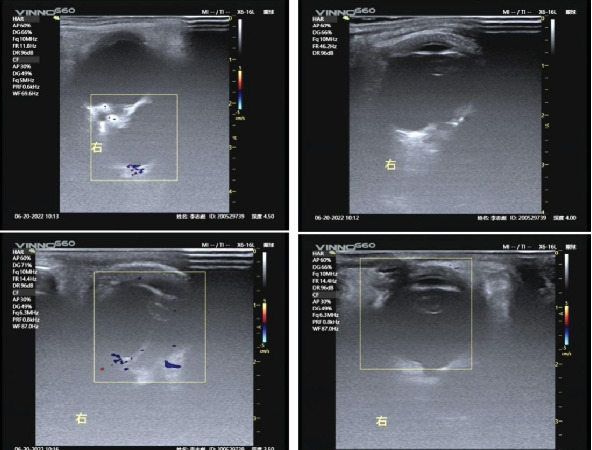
Doppler ultrasonography findings of the right eye. A large anechoic area is detected behind the wall of the eyeball, around which blood flow signals are detected.
